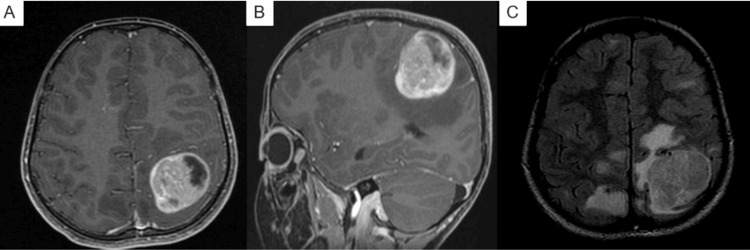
Radiologic findings on brain MRI (A, B) T1-weighted MRI with contrast shows a large ill-defined mixed cystic and solid lesion (4.3 × 3.5 × 3.5 cm) at the left parietal lobe, associated with surrounding white matter swelling. (C) T2-FLAIR image demonstrates multiple bilateral asymmetric subcortical white matter hyperintensities, the largest located at the posterior right parietal lobe.
